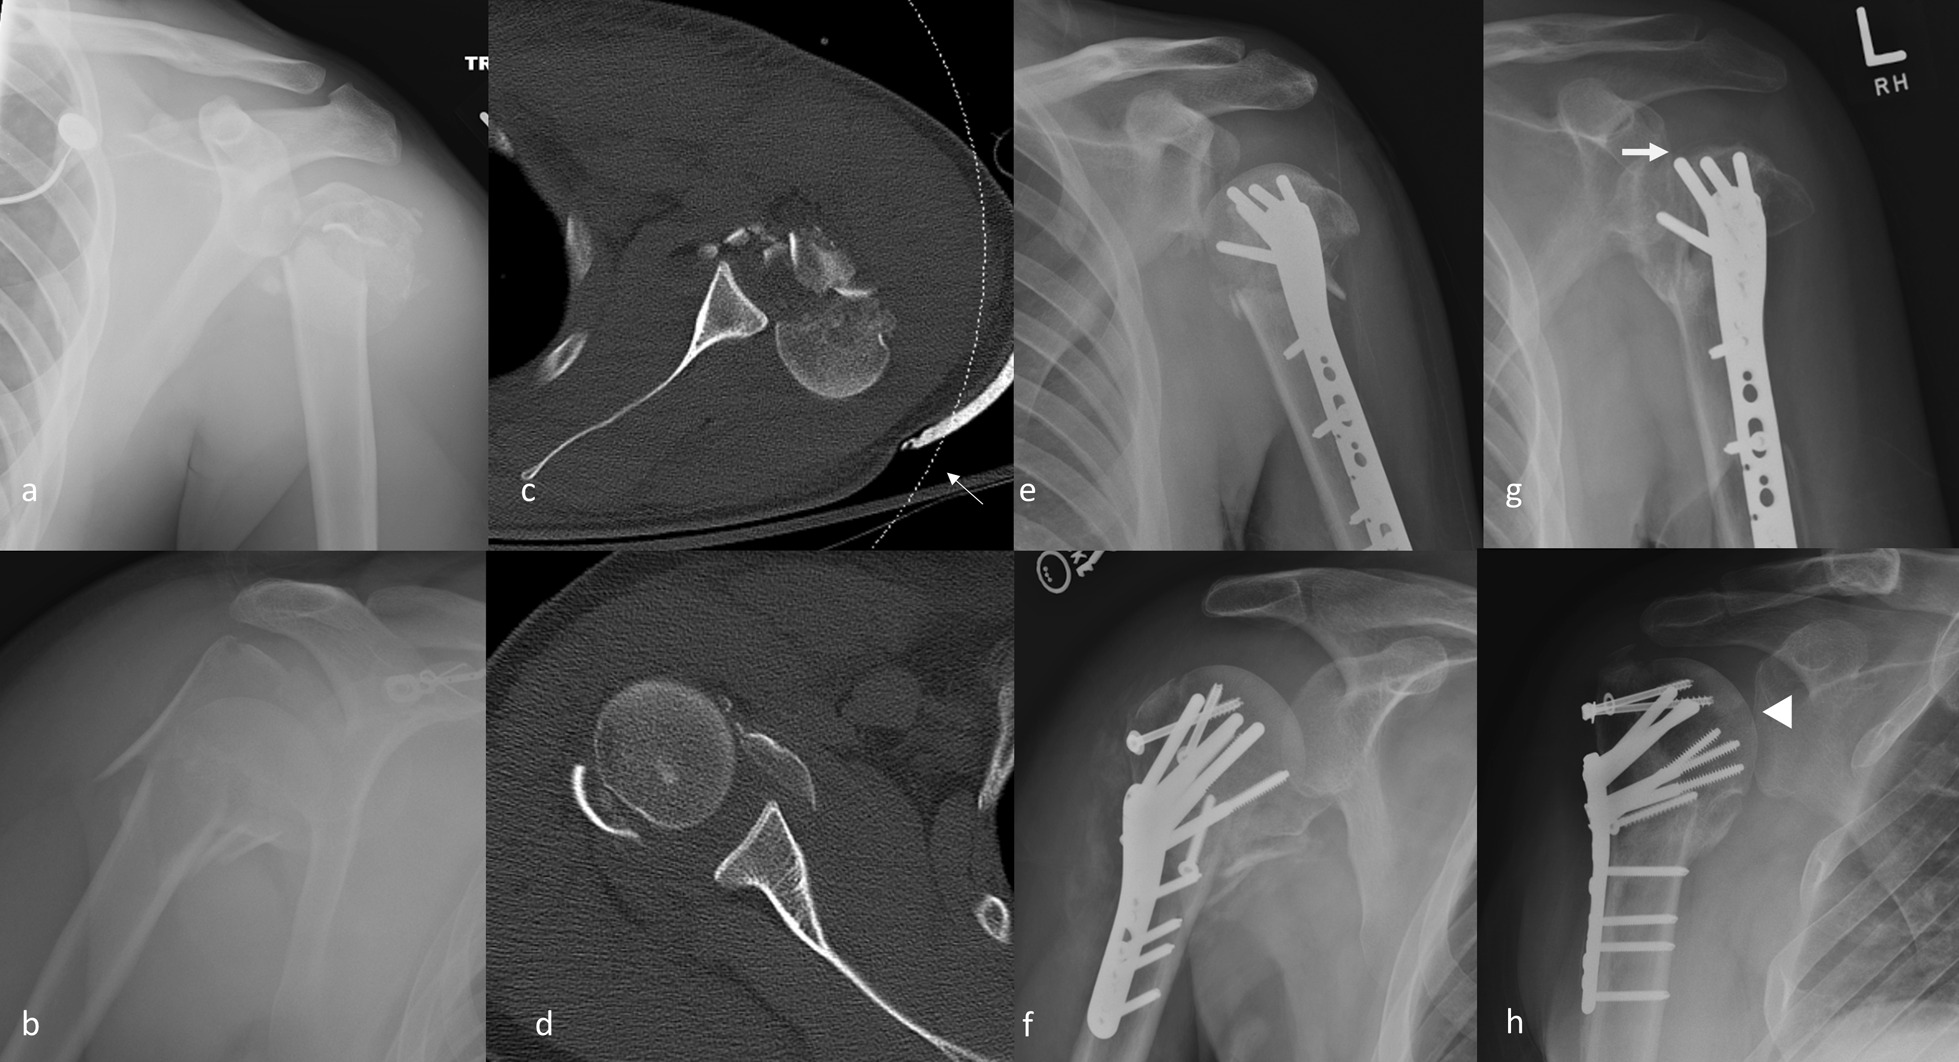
Initial radiographic and CT images with follow up imaging demonstrating AVN. Images from a 26-year-old male (top row, a, c, e, g) and a 36-year-old male (b, d, f, h) each with Neer four-part fractures were obtained including a, b initial frontal radiograph, c, d axial CT image, e, f post-ORIF radiograph and g, h evidence of osteonecrosis. g An attenuated linear shell of cortex with underlying lucency consistent with subchondral collapse due to AVN is present at 12 months after treatment (block arrow). h Subtle, circumscribed sclerosis adjacent to a focal lucency (block arrow) is consistent with AVN without collapse. The dashed line (c, thin arrow) indicates the outer margin of the 100 kVp source
